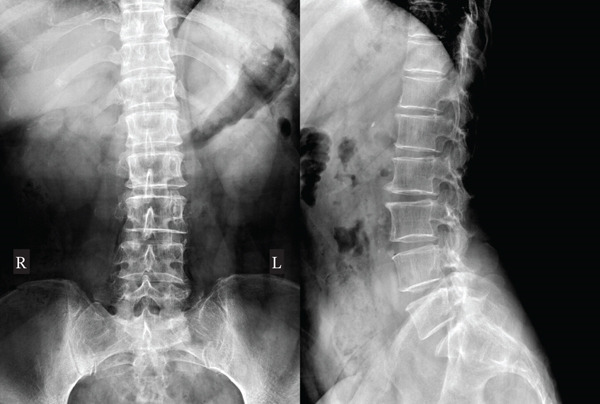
Anteroposterior and lateral radiographs of the lumbar spine.
